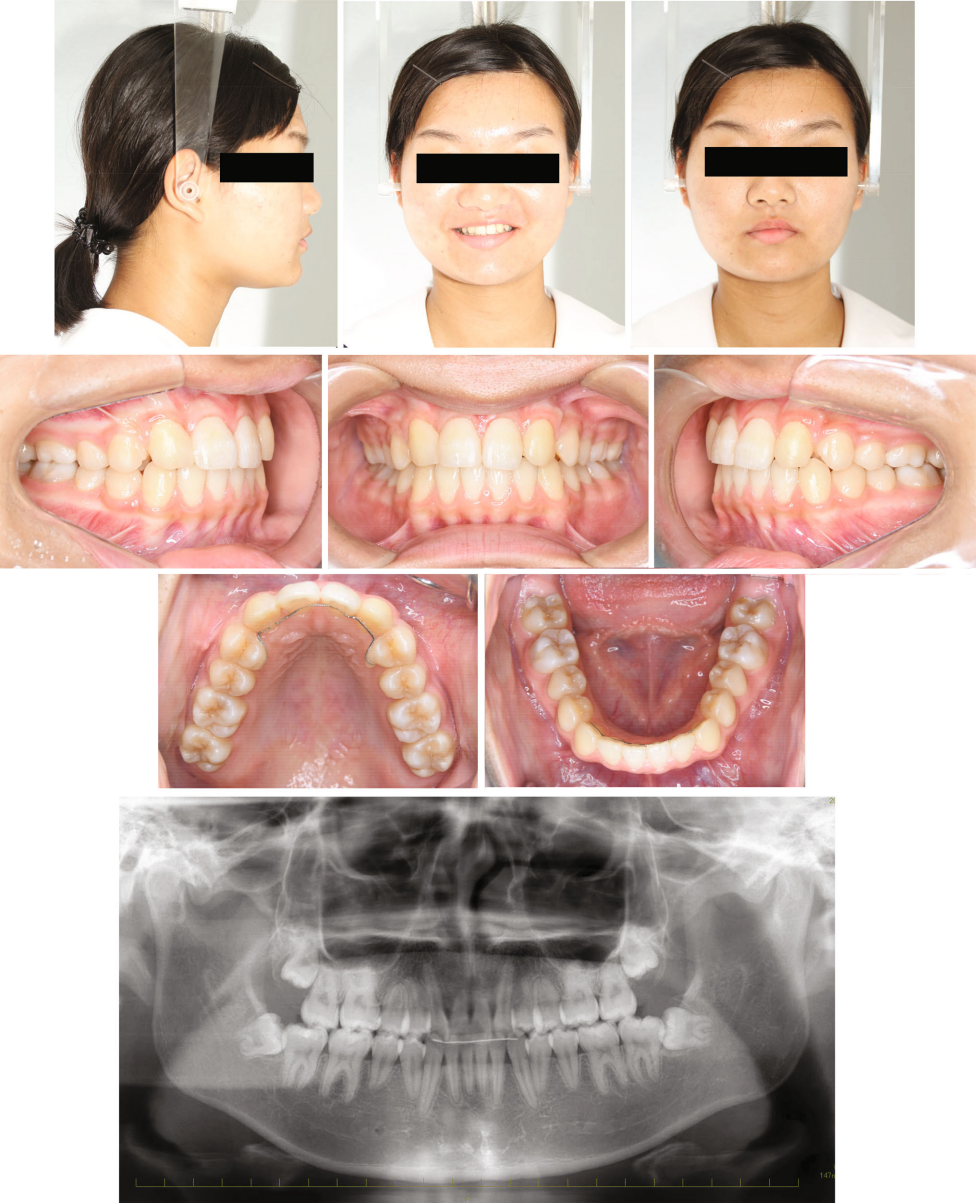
Posttreatment facial and intraoral photographs and panoramic radiograph.
